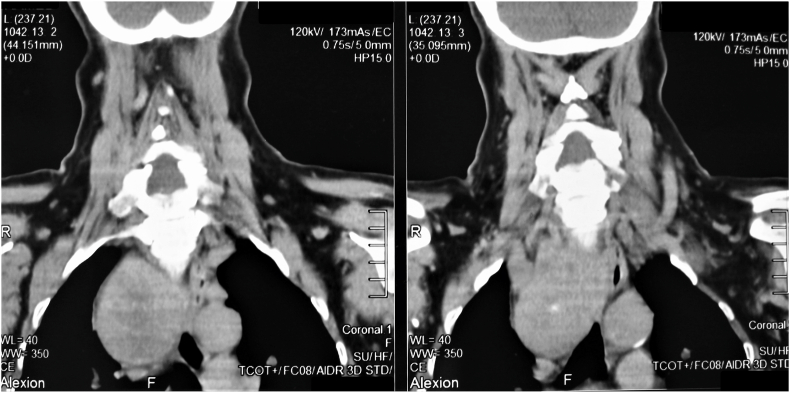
CT scan of a CMG extending below the level of the aortic arch and reaching the level of carina on the right side.
